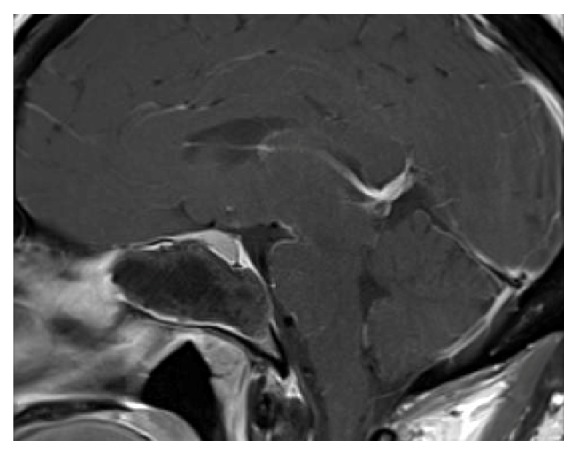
MRI of brain with sagittal view demonstrating expansile lesion centered in sphenoid sinus. The area with the signal void represents fungal debris. There is noticeable mass effect upon the sella with superior deviation of the pituitary gland and kinking of the infundibulum.
